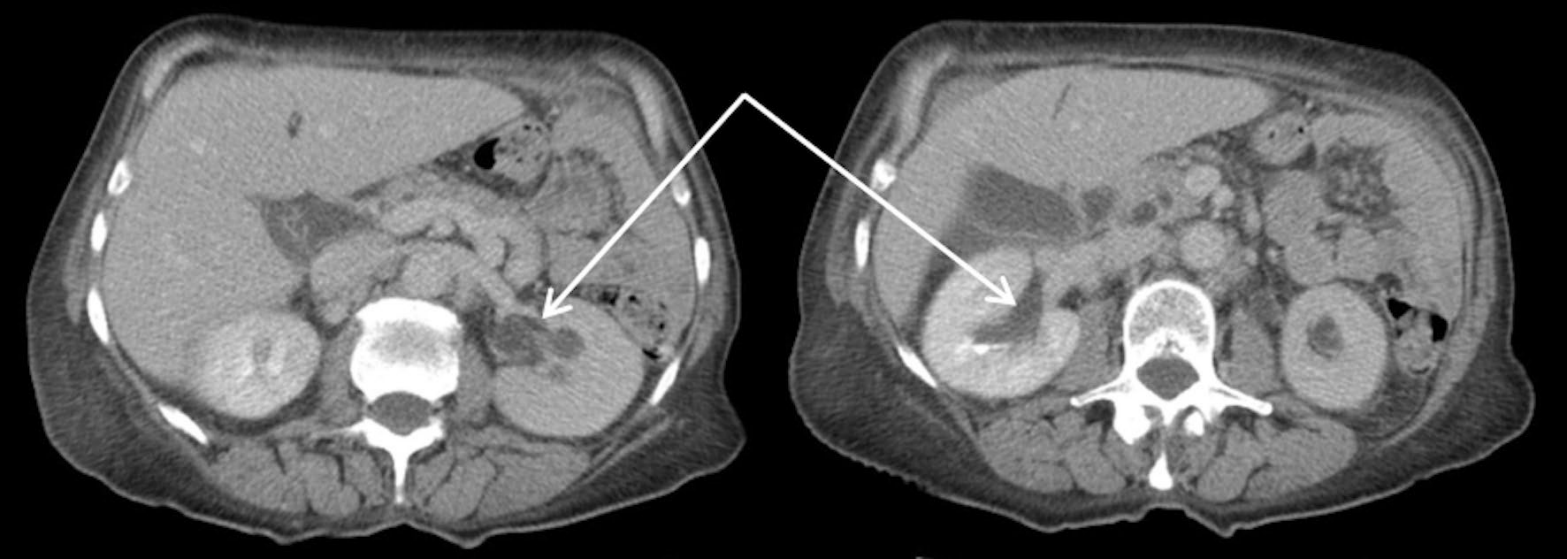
CT scan showing hydronephrosis of the left and right kidneys
